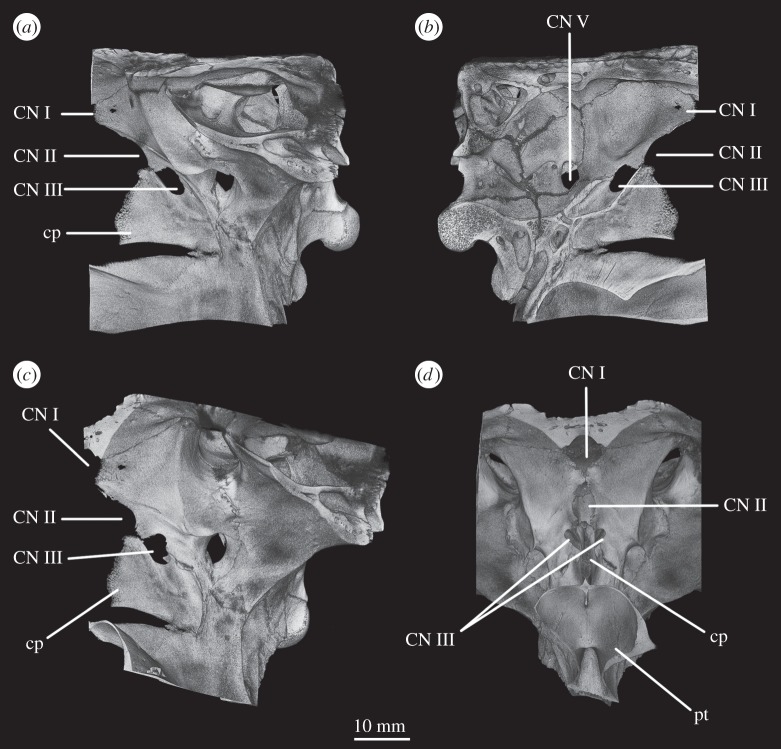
CT reconstructions of braincase of *Osteolaemus tetraspis* (ZMB 23467) in (*a*) left lateral, (*b*) left medial, (*c*) left anterolateral, and (*d*) anterior views. For abbreviations, see table 1.
